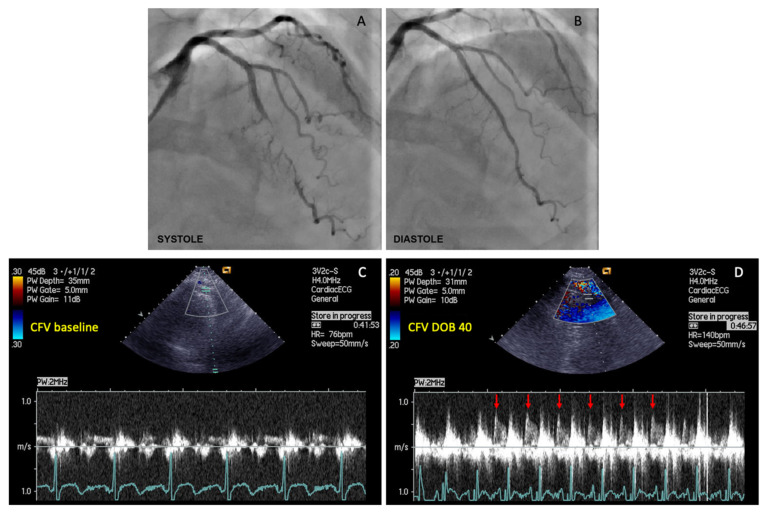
The example of coronary flow velocity reserve (CFVR) measurements obtained by transthoracic Doppler echocardiography (TTDE) in the left anterior descending (LAD) artery distal to the myocardial bridging (MB), before and during iv. infusion of high-dose dobutamine (40 µg/kg/min). (A) Coronary angiography revealed myocardial bridging (MB) with severe intramyocardial LAD segment compression (>90% diameter stenosis) during systole; (B) Coronary angiography showed a significant decompression of intramyocardial LAD segment during diastole in the same patient; (C) CFV measurement under basal conditions (CFV baseline), and (D) CFV measurement at peak dobutamine dose (CFV DOB 40). The heart rate under basal conditions was 76 bpm, while during peak dobutamine infusion was 140 bpm (delta-HR 64 bpm). Coronary flow velocity reserve equals 2.96 (CFV DOB 40/CFV baseline = 0.77/0.26 = 2.96). Red arrows showing characteristic diastolic CFV profile during peak dobutamine infusion in the LAD distal to the MB. This phenomenon is characterized by an abrupt acceleration followed by rapid deceleration of the CFV at early-diastole and flow plateau during mid-to-late diastole (“finger-tip” phenomenon). CFV = coronary flow velocity; DOB 40 = dobutamine at 40 µg/kg/min.
